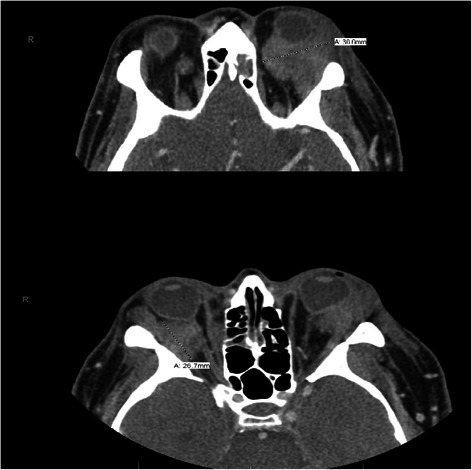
Computed tomography scan of the head showing increase in size of the orbital masses, measuring 3.7 cm on the left and 2.7 cm on the right, causing a mass effect on the optic nerves
